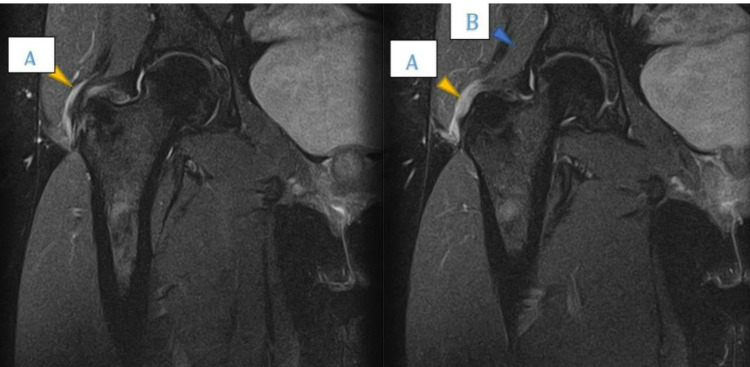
Magnetic resonance images of the right hip (T2-weighted). (A) High-intensity signal at the superolateral aspect of the greater trochanter, showing a partial tear of the gluteus minimus at its insertion. (B) Gluteus minimus muscle.
